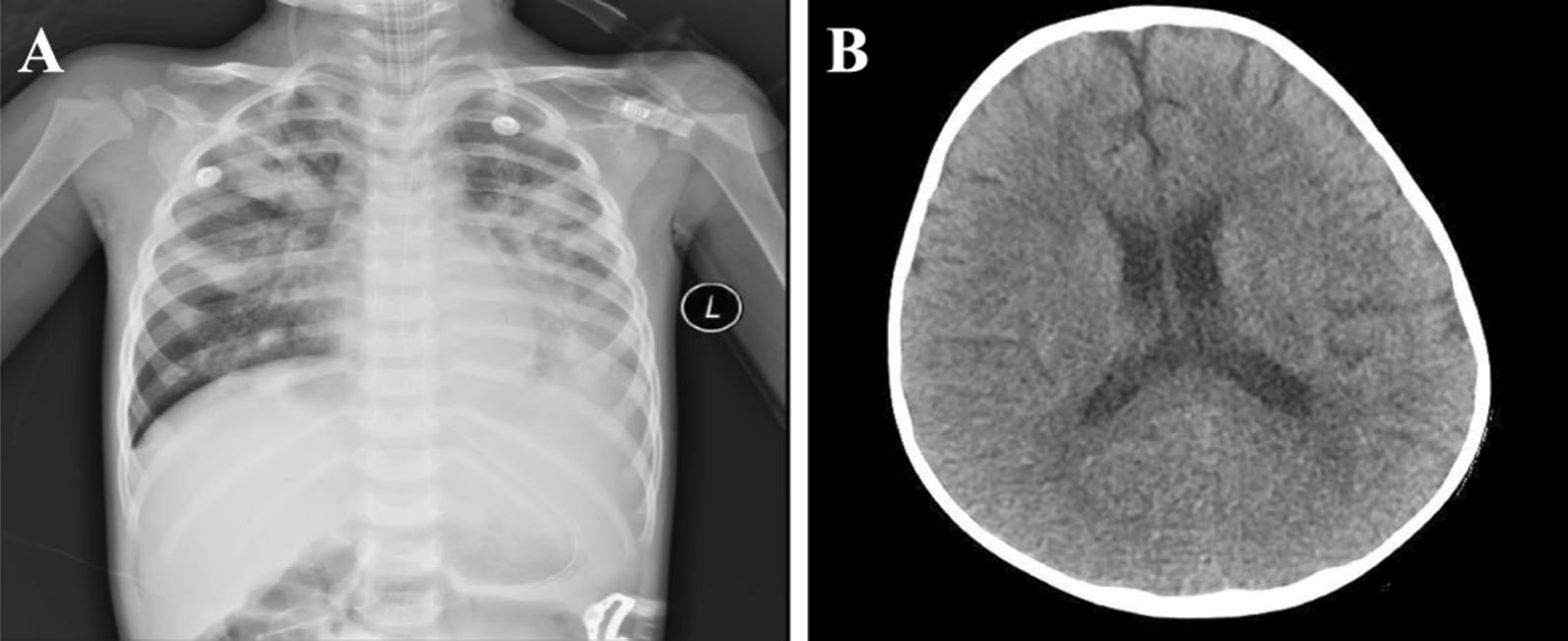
A Chest computed tomography image obtained 5 days after admission. B Cerebral computed tomography image obtained 5 days after admission
